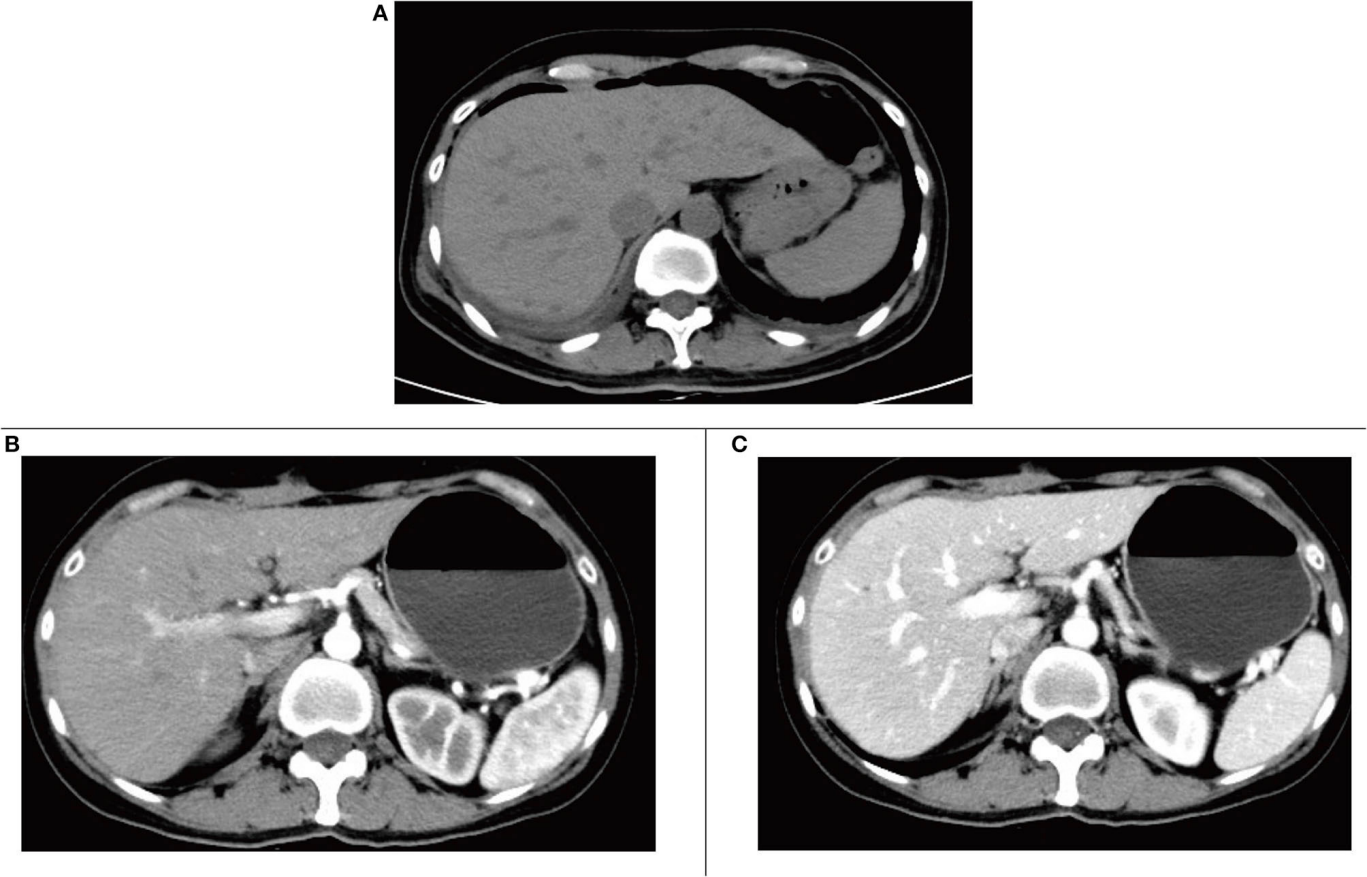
(A) Computed tomography (CT) plain scan after surgery. (B) The arterial phase of enhanced CT scan 2 months after surgery. (C) The portal phase of enhanced CT scan at postoperative 2 months.
